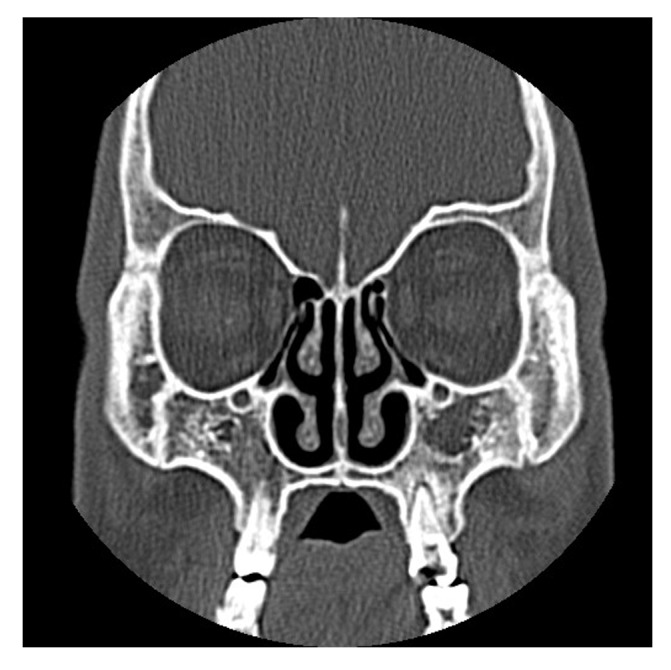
Paranasal Sinus CT, Coronal Section, Indicating Aplasia of the Maxillary Sinus and Minimal Pneumatization in the Ethmoid Cells.
